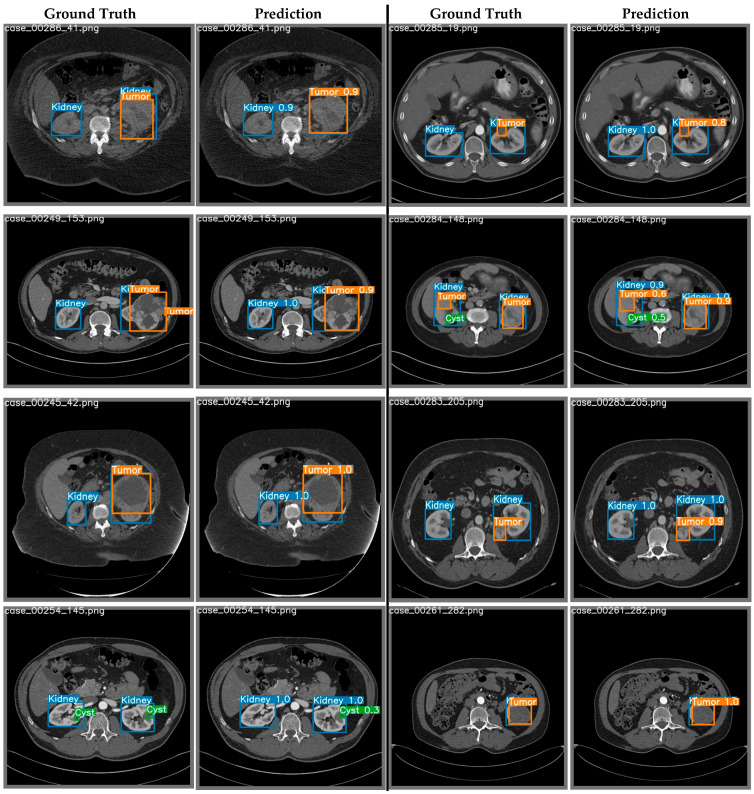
Example ground-truth labels vs. prediction plots from the best performing YOLOv7 model. Shown is the detection of kidney, tumor, and cyst ROIs from CT slices (displayed for qualitative evaluation).
